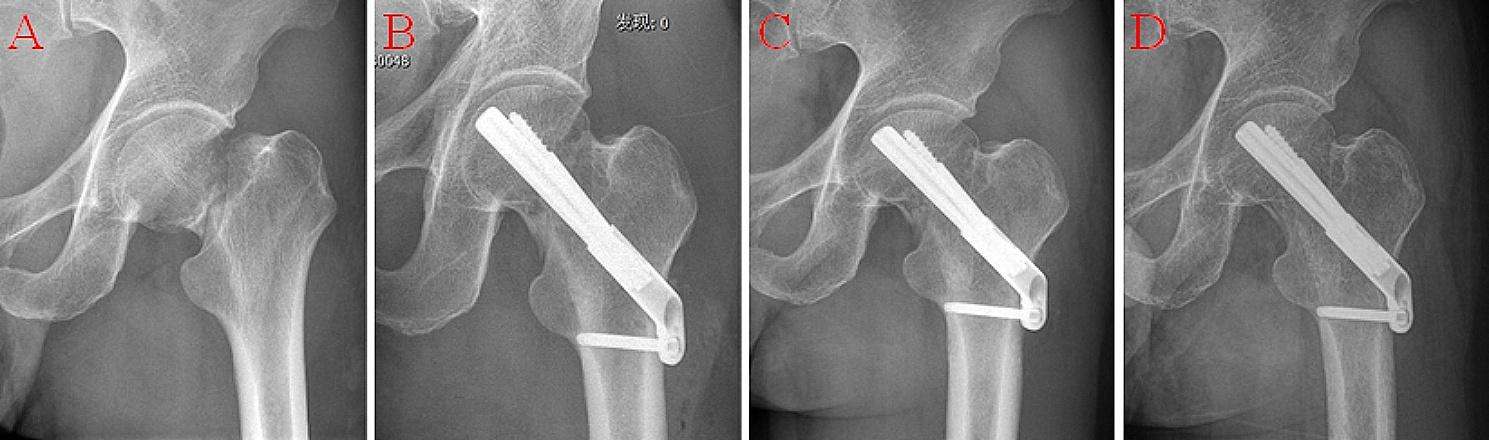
Radiograph of the femoral neck after the surgery: (A) preoperative, (B) postoperative, (C) 3 months postoperative, (D) 6 months postoperative
